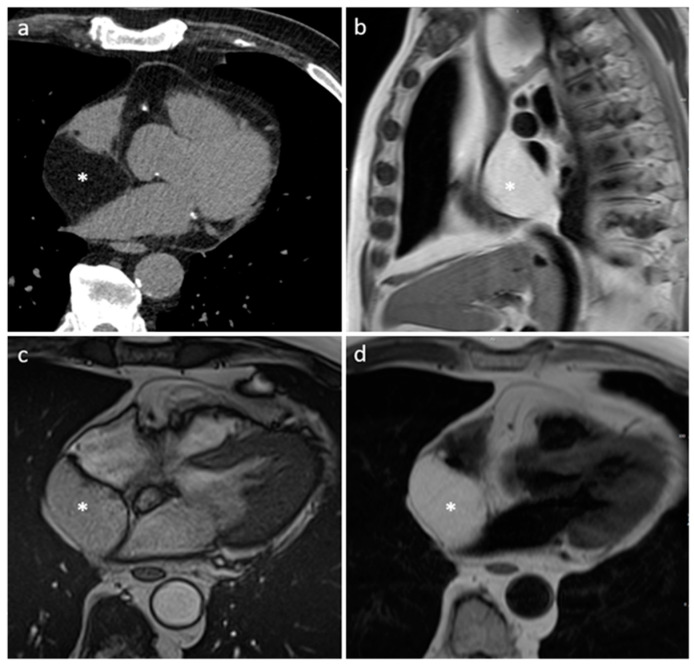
Right atrium (*) posterior wall capsulated ovoid fatty mass in CT scan (a), T1-weighted images (b,d) and cine (c) MR images.
